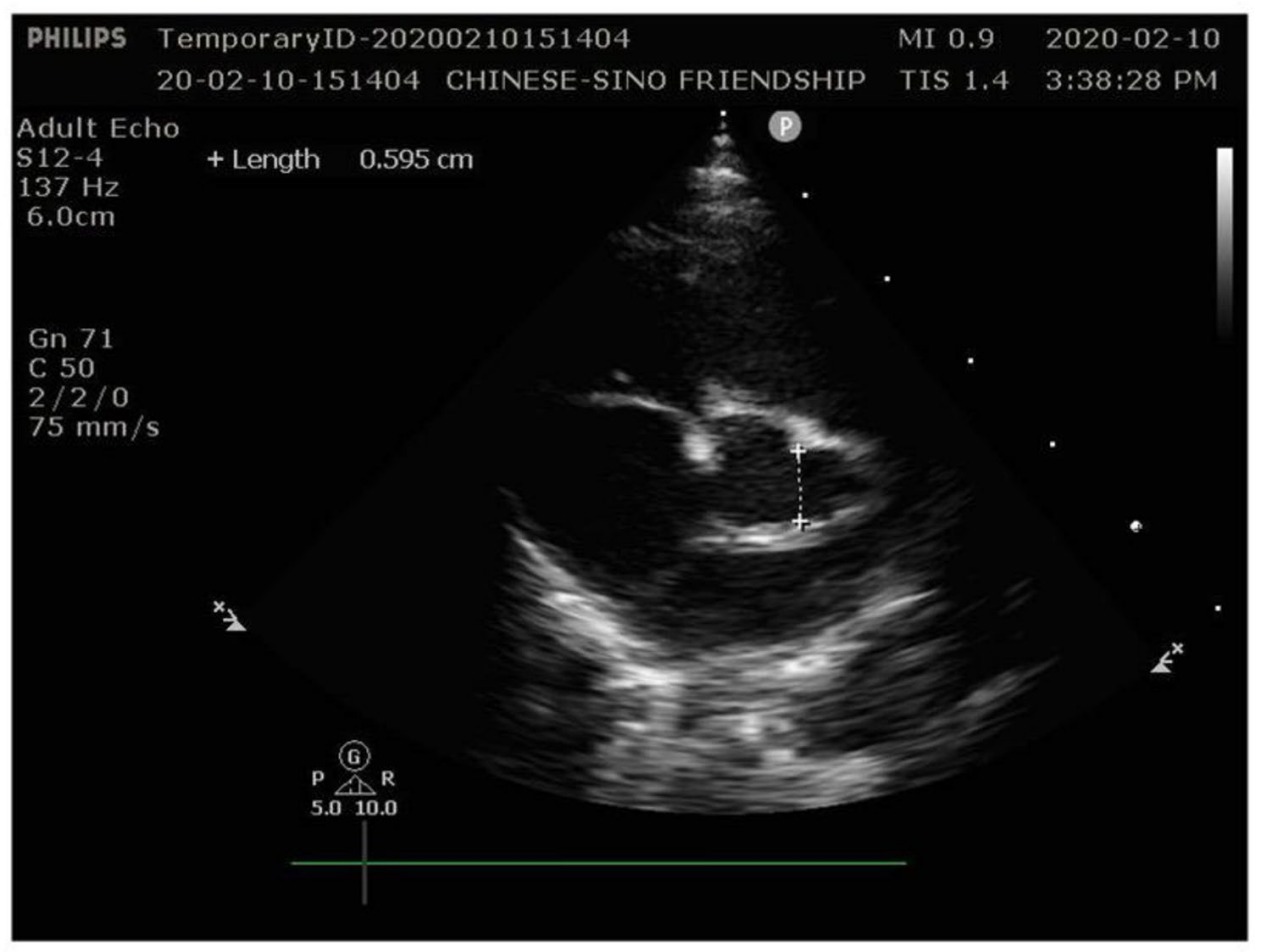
Echocardiography on 15th day after birth revealed coronary artery aneurysms in the left coronary artery
